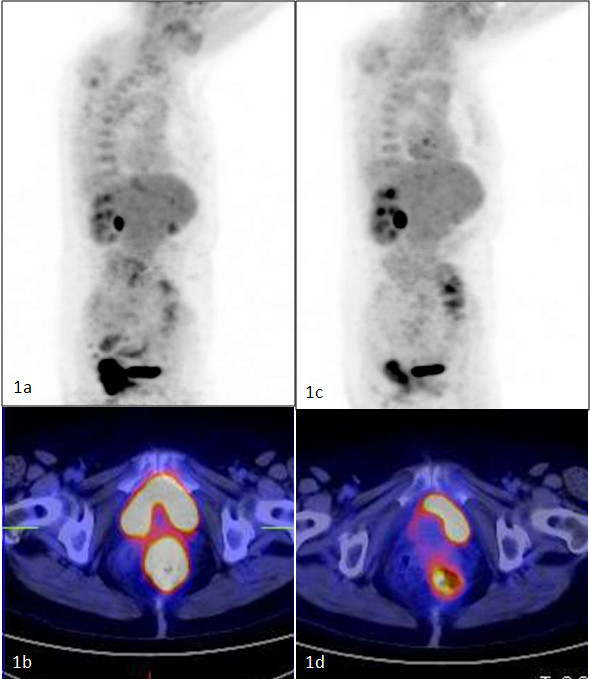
Partial metabolic response to CRT. 2A: Pre-CRT study - Intense rectal FDG uptake. 2B: Pre-CRT study - axial PET-CT images showing hypermetabolic rectal mass. 2C: Post-CRT study - Tumour volume is reduced but considerable tumour uptake is still present. 2D: Axial PET-CT images showing rectal mass in CT images with FDG uptake in PET images.
